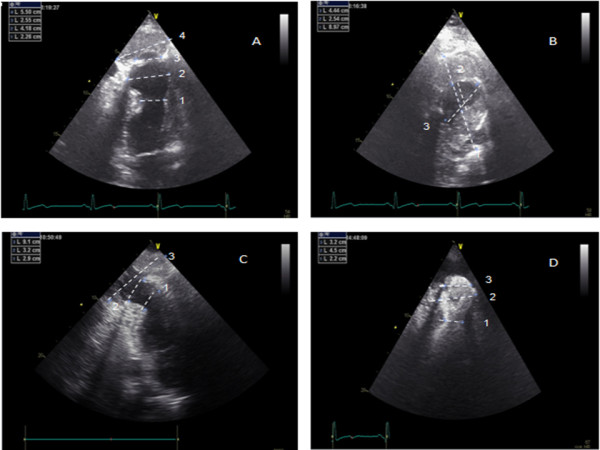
Transthoracic echocardiogram revealing the pseudoaneurysm with a large thrombus inside. A- Four chamber view (1-aneurysm neck; 2-aneurysm maximum diameter; 3- pseudoaneurysm neck; 4- pseudoaneurysm maximum diameter), B- Parasternal short axis view at apical level (1- pseudoaneurysm maximum diameter; 2 and 3- pseudoaneurysm neck) C- Two chambers view in systole with the maximum diameter of the neck [2] and the maximum internal diameter of the pseudoaneurysm [3]. D- Four chamber view using contrast (1-aneurysm neck; 2- aneurysm maximum diameter; 3- pseudoaneurysm neck).
